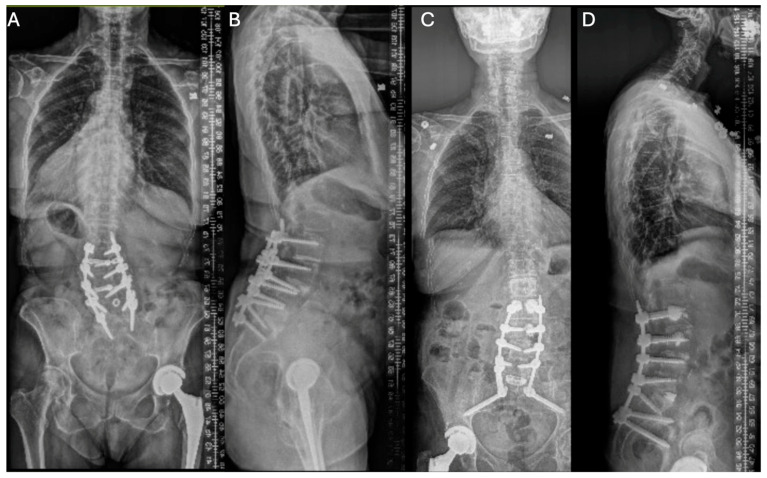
Case 1: pre- versus postoperative standing X-rays. Preoperative AP (A) and lateral (B) standing scoliosis X-rays showing a coronal deformity, a mild sagittal imbalance, and a loss of lumbar lordosis. Postoperative AP (C) and lateral (D) images showing an improved coronal deformity, lumbar lordosis, and a sagittal imbalance.
